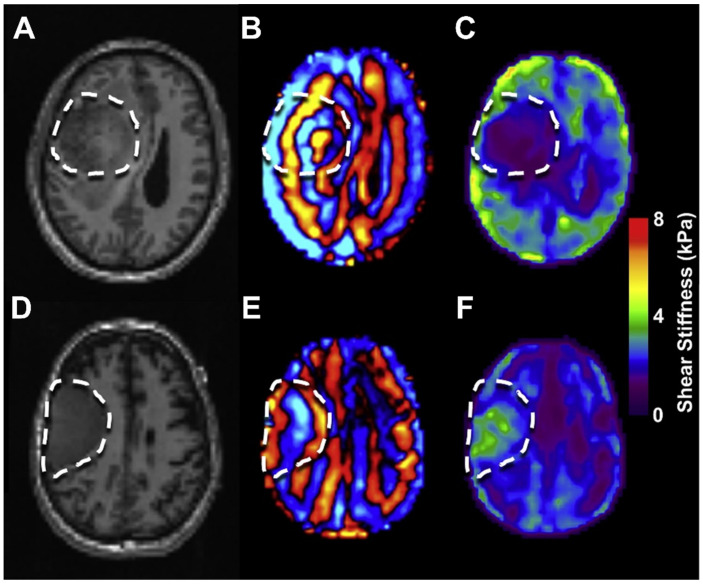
Tumor stiffness differences for two patients with meningiomas. T2-weighted anatomical images (A,D), curl wave images (B,E) and elastograms (C,F). The first patient (A–C) had a substantially softer meningioma than the second one (D–F). In the first example, the shear wavelength is much shorter than in the second one. Figure adapted with permission from Ref. [108]. 2015, Pepin K.
